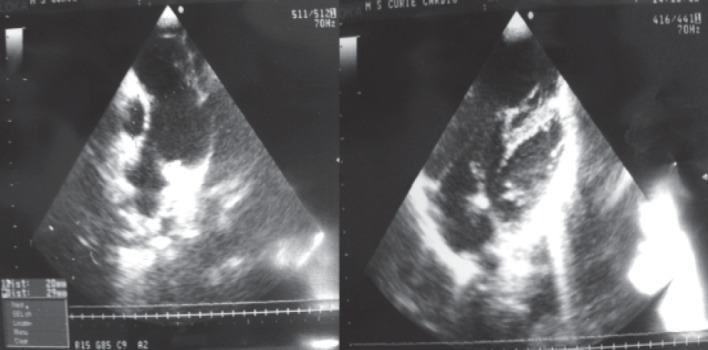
Transthoracic ultrasound examination after surgery, at the age of 13 years, showing dilated right heart, requiring repeating echocardiography exam after 6 months
